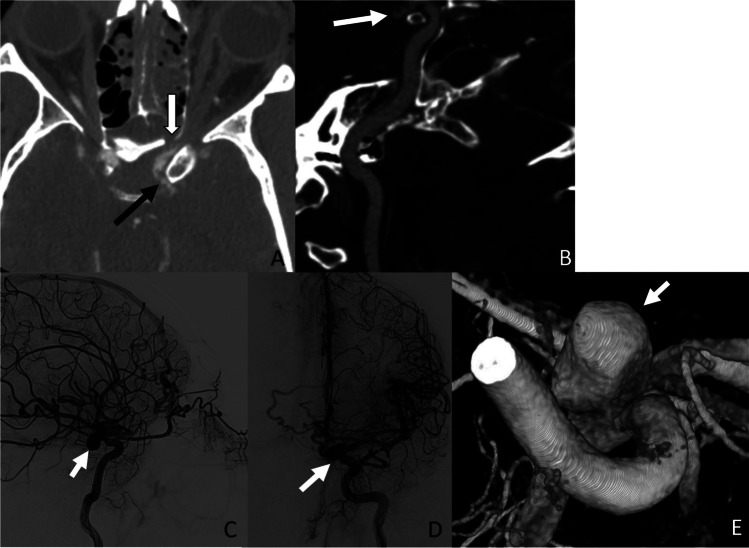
Post-traumatic aneurysm. A 45-year-old male presented with severe craniofacial trauma involving the frontobasal distribution after an ATV rollover. The initial CTA neck demonstrated vessel irregularity and non-flow limiting stenosis along the left supraclinoid ICA adjacent to the sphenoid sinus wall fracture (A and B). The left ICA injections in the lateral, frontal projections, and 3D reformats on the follow-up DSA demonstrated the development of a medially projecting ICA sidewall pseudoaneurysm (C, D, and E)
